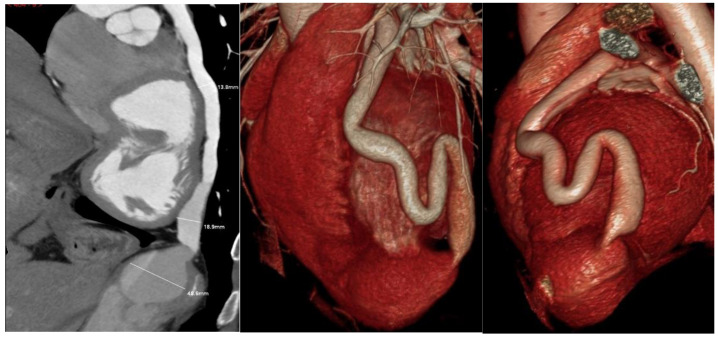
CT angiography revealing large fistula communicating with aneurysmal sac.
